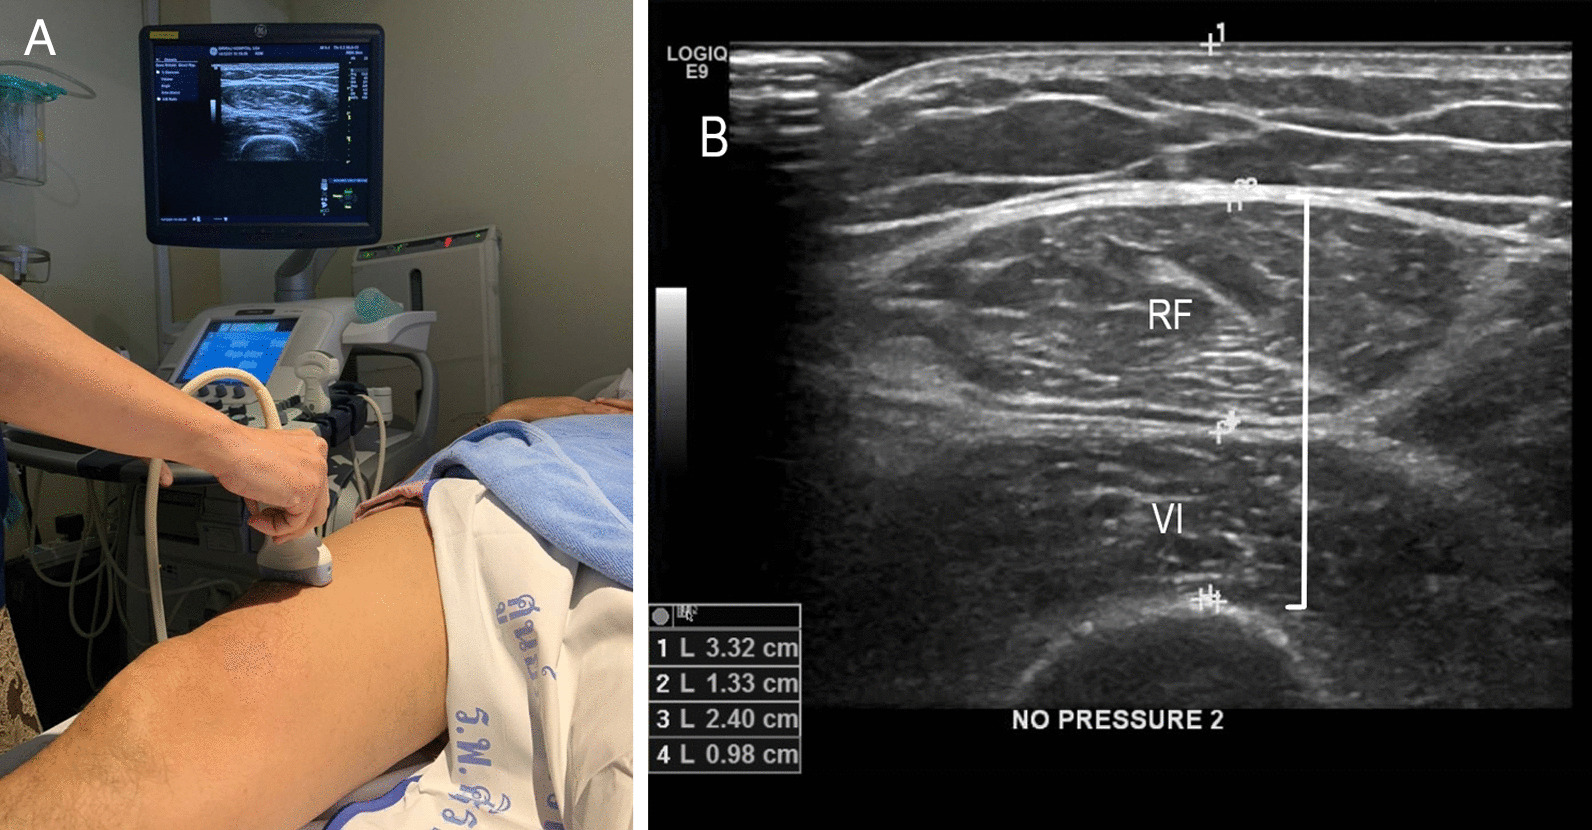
Ultrasonography was used to measure thigh muscle thickness. The locations of measurement were the depth of the right quadriceps muscle at one-third and one-half of the total distance from the top of the patella to the iliac crest (A). The depth of the right quadricps muscle composed of the rectus femoris and vastus intermedius (B). Two readings were obtained for each point: a compression reading and a feather weight reading. Abbreviation: RF, rectus femoris; VI, vastus intermedius
